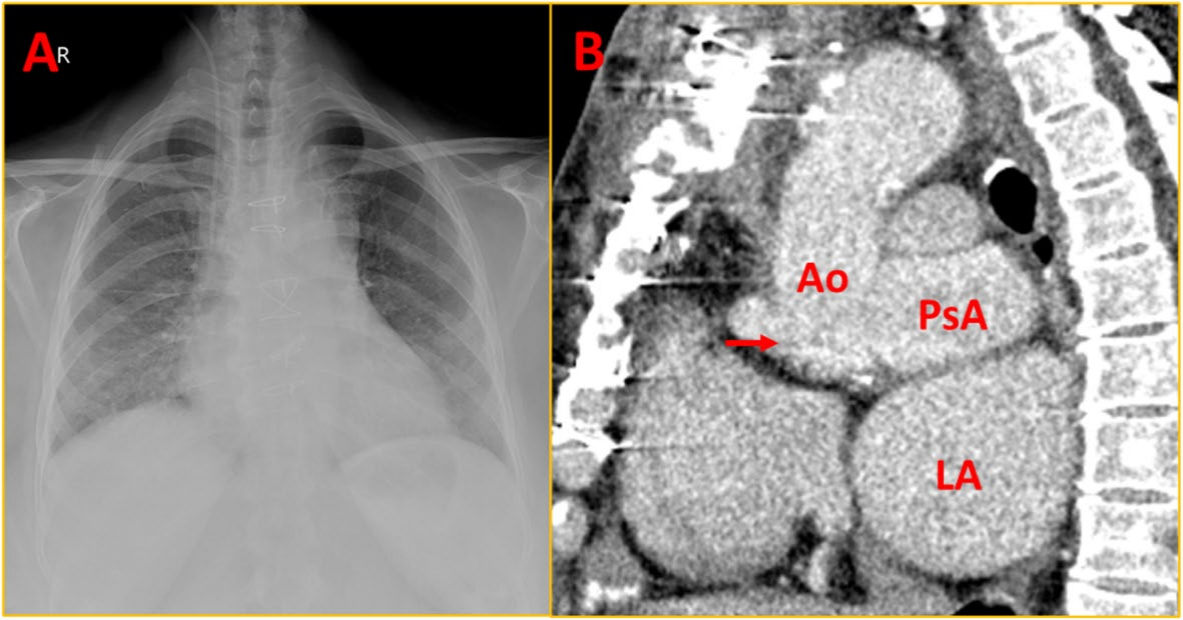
Chest X-ray and Cardiac CT following pseudoaneurysm repair. A Chest X-ray showing near complete resolution of mediastinal mass after surgery. B Repeat cardiac CT showing persistence of aortic root pseudoaneurysm and resolution of ascending aortic pseudoaneurysm. Arrow indicates the aortic valve level (the beam hardening artifact from prosthetic valve). CT = computed tomography; PsA = pseudoaneurysm at aortic root; Ao = aorta; LA = left atrium
